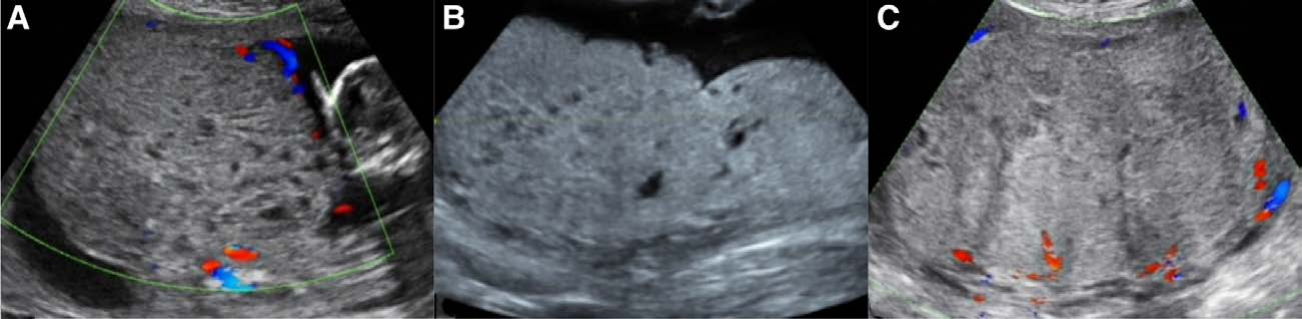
Ultrasound findings of placental mesenchymal dysplasia (PMD). (A) Sonographic image at 15 + 5 weeks’ gestation showing 106 × 68 mm^2^ mixed echo above placenta, partial anechoic area inside, honeycomb like. (B) Sonographic image at 25 + 5 weeks’ gestation showing 142 × 101 mm^2^ mixed echo above placenta, partial anechoic area inside, honeycomb like. (C) Sonographic image at 30 weeks’ gestation showing 144 × 130 × 89 mm^3^ mixed echo above placenta, partial anechoic area in the inner part and point strip blood flow in the inner part.
